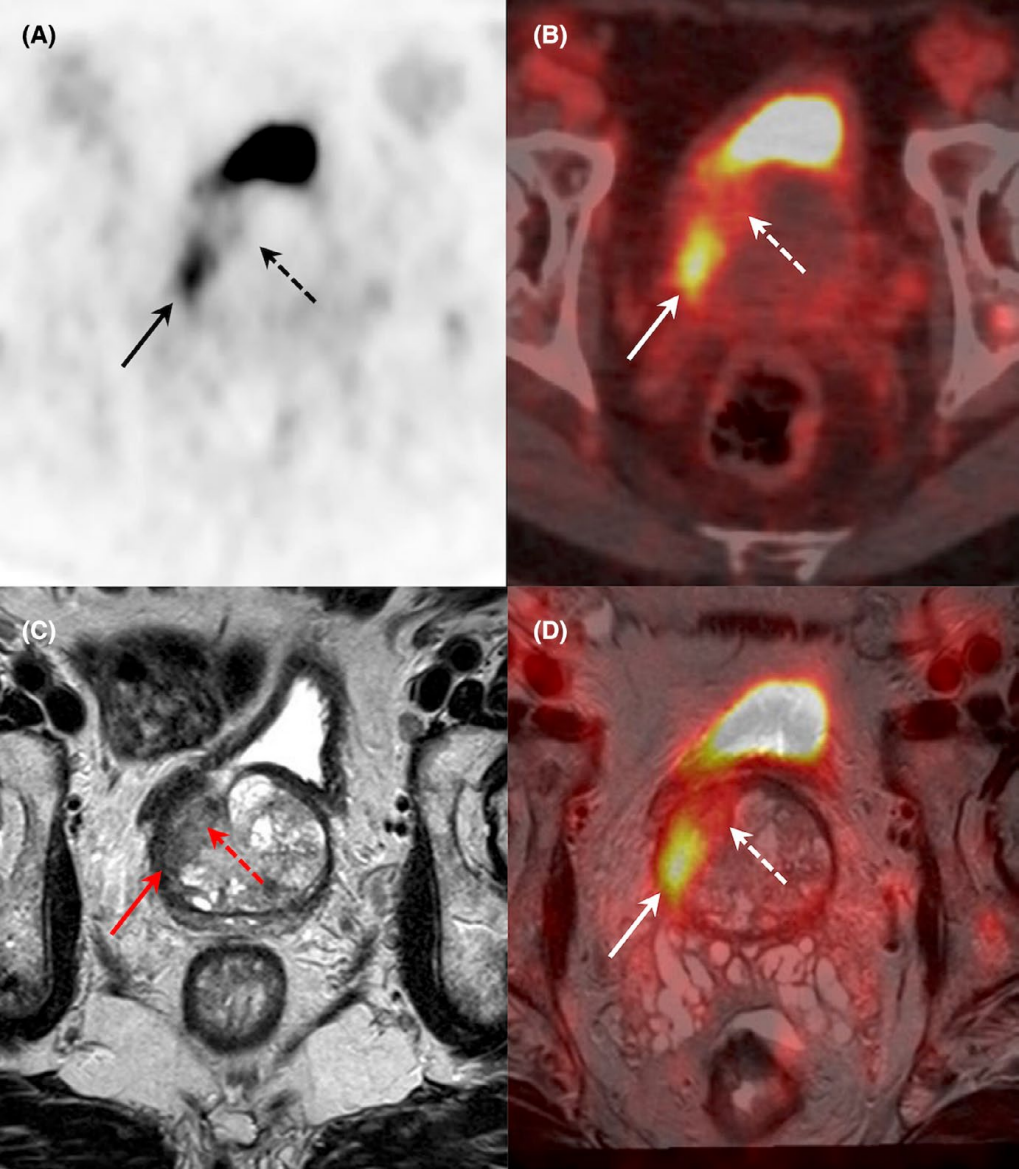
Results of [^18^F]DCFPyL PET/CT and MRI imaging (axial PET (A), PET/CT (B), T2‐weighted MRI (C), PET/MRI fused images (D)) in a patient with prostate cancer biological suspicion (PSA: 18.6 ng/mL). MRI revealed a tumoral lesion of 25 × 21 × 18 mm in the posterior part of the right transitional zone (MRI PI‐RADS: 5). Intense [^18^F]DCFPyL focal uptake (SUVmax: 12) was shown exclusively in the posterior part of the lesion. Pathological examination after biopsy confirmed the presence of acinar adenocarcinoma, Gleason score: 7 (4 + 3). After surgery, tumour was localized only in the PET‐positive lesion, with extracapsular invasion.
